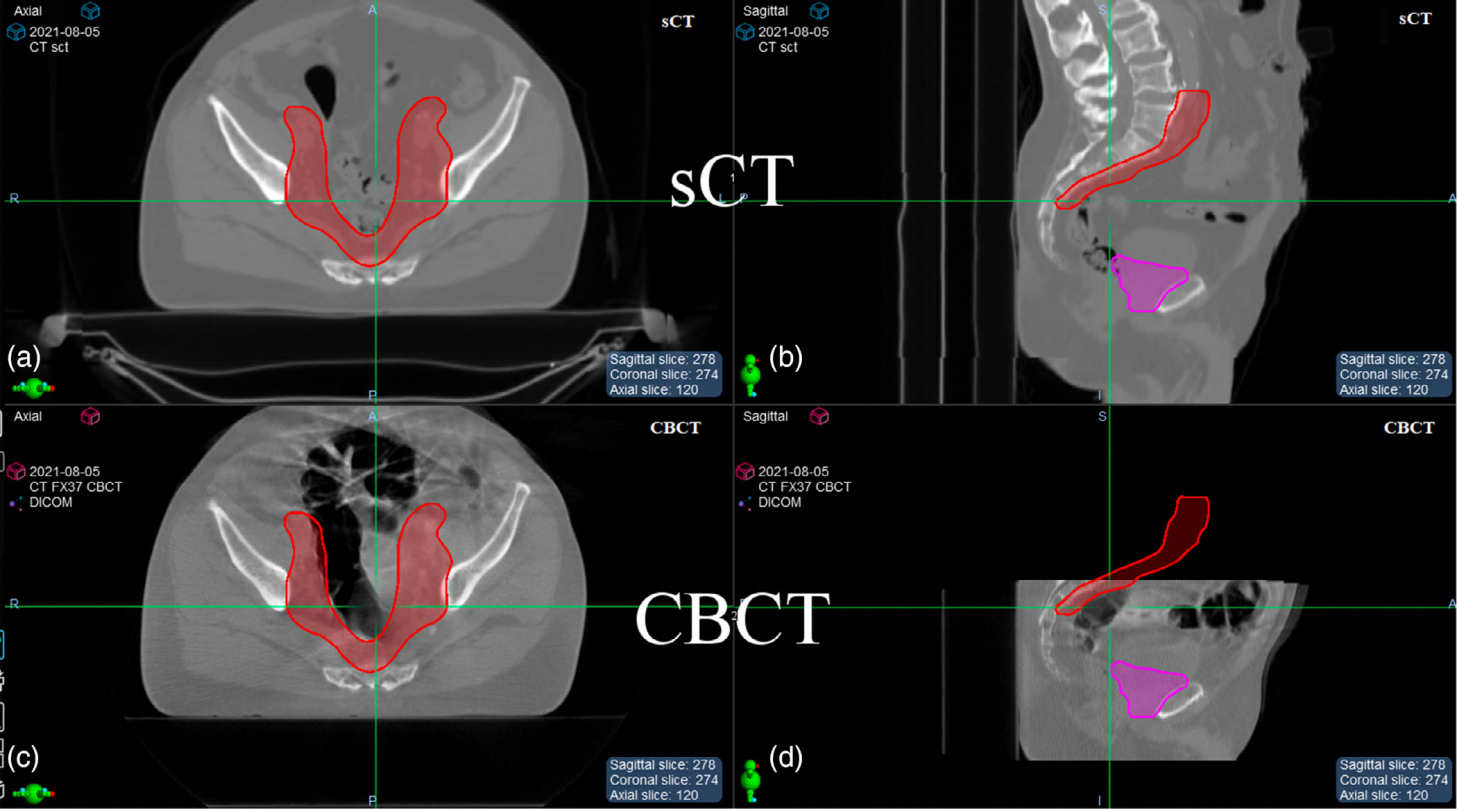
CBCT and respective sCT images for P1‐FX37 showing the differences in abdominopelvic air cavities. (a) sCT axial. (b) sCT sagittal. (c) CBCT axial. (d) CBCT sagittal. PTV6840 (magenta) and PTV4500 (red) were overlaid on both images to show the proximity and overlap with the uncorrected air volume.
